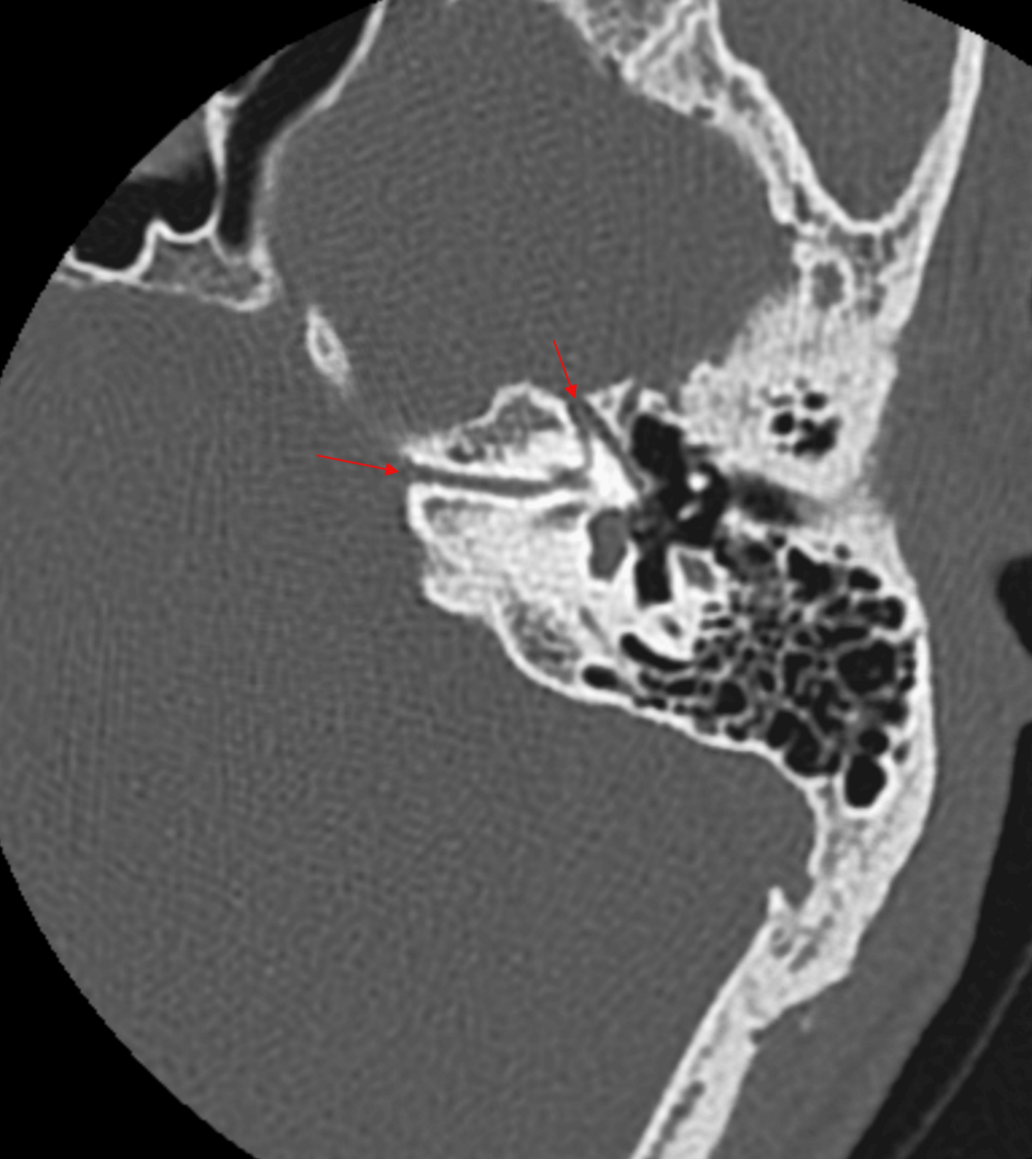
Axial oblique reformatted HRCT image in the bone window showing antero-superior bony canal in continuation with the labyrinthine segment of the left facial canal (red arrows)
